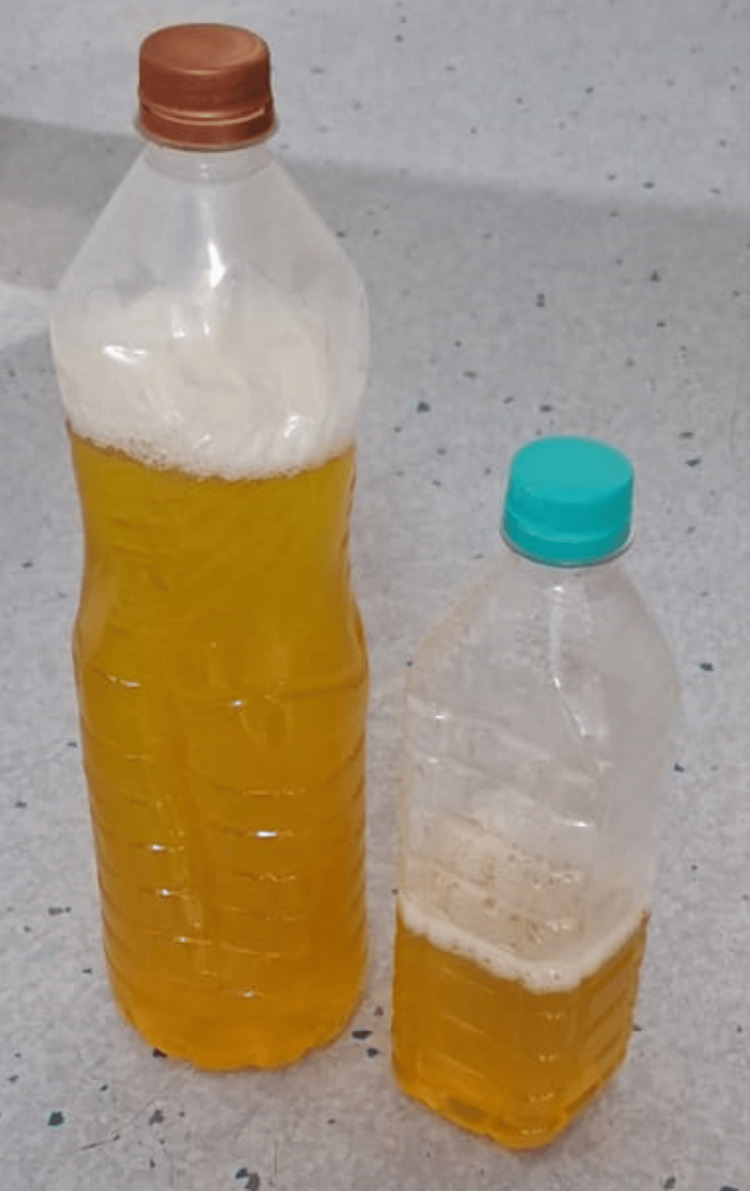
Straw-coloured pleural fluid removed through ultrasonography (USG)-guided thoracocentesis
